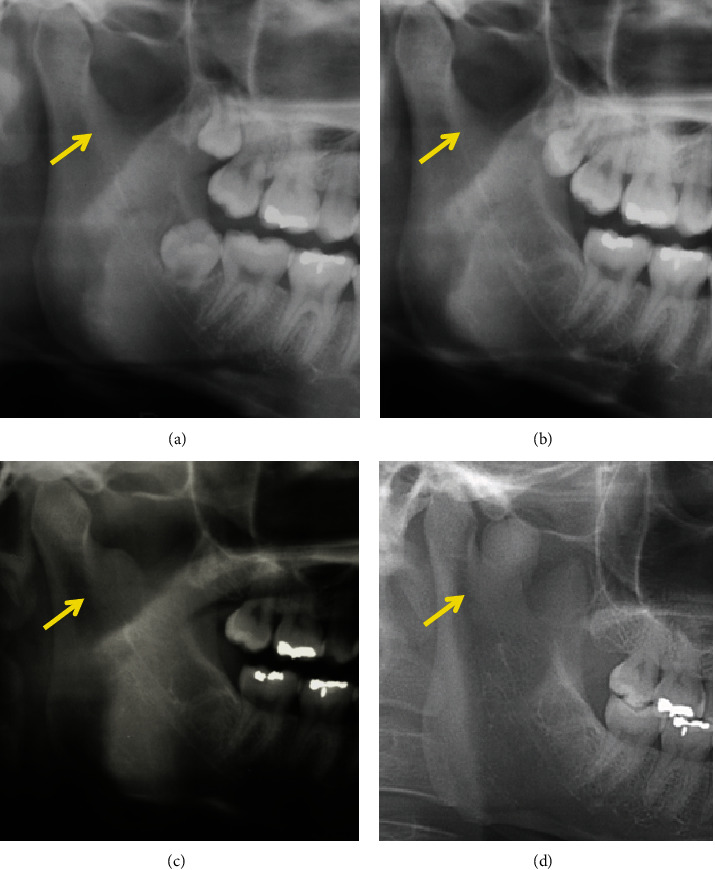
Time course of osteoma in the right mandibular notch by panoramic radiography. (a) At the age of 18, no osteoma was found in the right mandibular notch. (b) At the age of 21, no osteoma was found in the right mandibular notch. (c) At the age of 26, a bony bulge was found in the right mandibular notch. (d) At the age of 38, an oval radiodense mass was observed in the right mandibular notch.
